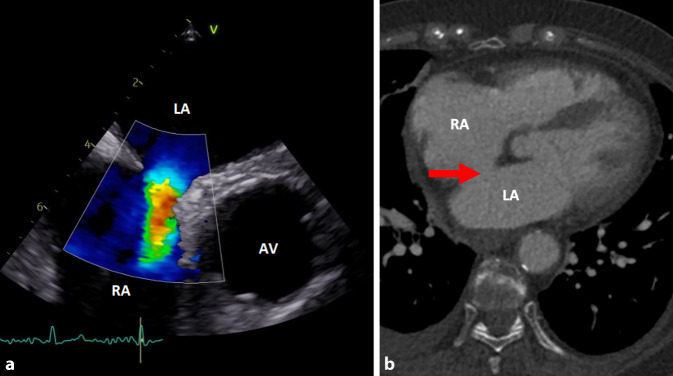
a Darstellung des ASD in der TEE. Der aus dem Defekt des Septums interatriale resultierende Shunt zwischen linkem (*LA*) und rechtem Vorhof (*RA*) ist sehr gut erkennbar. Rechts im Bild befindet sich die Aortenklappe (*AV*). b Auch in der CT lässt sich der ASD (*Pfeil*) darstellen
